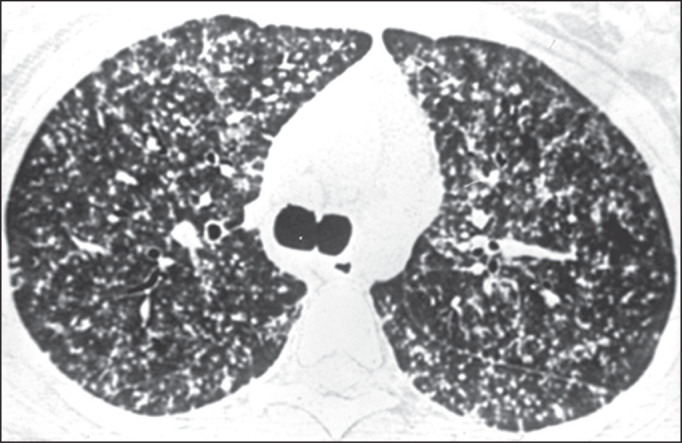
CT of a 39-year-old man, showing small pulmonary nodules with a
